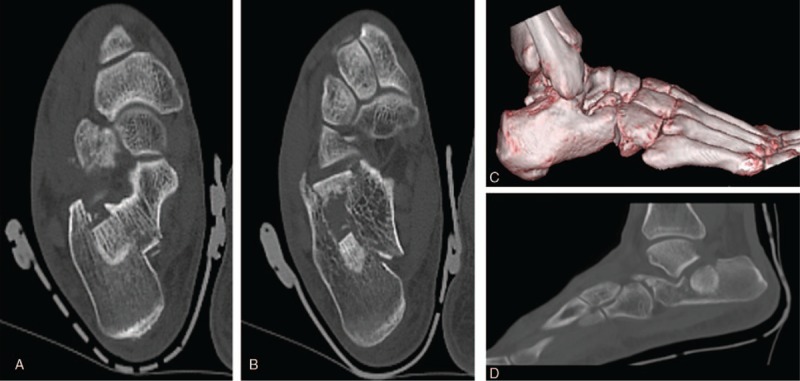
(D) CT scan showed collapsed calcaneal fracture in the middle of posterior facet. (A–C) The lateral wall of calcaneus was intact.
